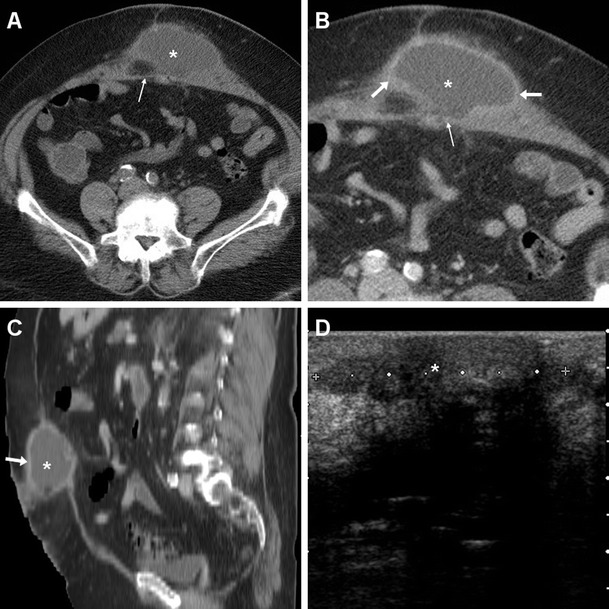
Postoperative abscess following recent open repair of post-laparotomic hernia in a 77-year-old female, clinically heralded by hard-consistency and inflamed swelling at the anterior abdominal wall. Unenhanced (a), post-contrast (b) axial and sagittal (c) CT images showed a sizeable (12x4 cm) collection (*) abutting the anterior aspect of the PM (thin arrows), with 16–18 Hounsfield units (HU) internal attenuation and thick irregular peripheral enhancement (arrows in b, c). The corresponding, unspecific sonographic appearance (d) was a poorly demarcated hypoechoic region (calipers) with posterior acoustic shadowing. The abscess resolved with percutaneous drainage and antibiotics
